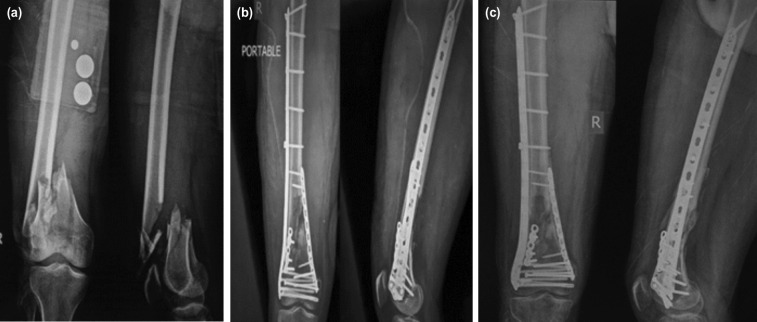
(a) Pre-operative radiograph (b) Immediate post-operative radiograph, (c) six months post-operative radiograph.
